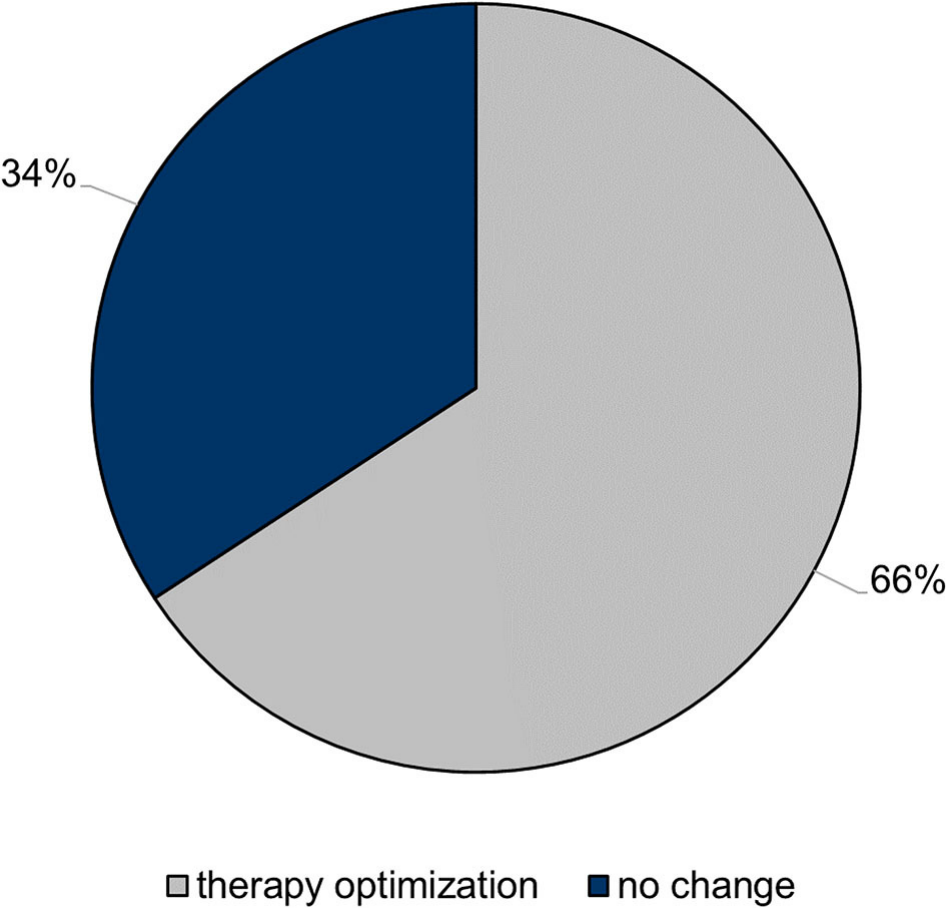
Impact of follow-up coronary CT angiography on patient management. In a relevant proportion of the study population, the therapeutic regimen was adjusted due to the results of the follow-up coronary CT angiography.
